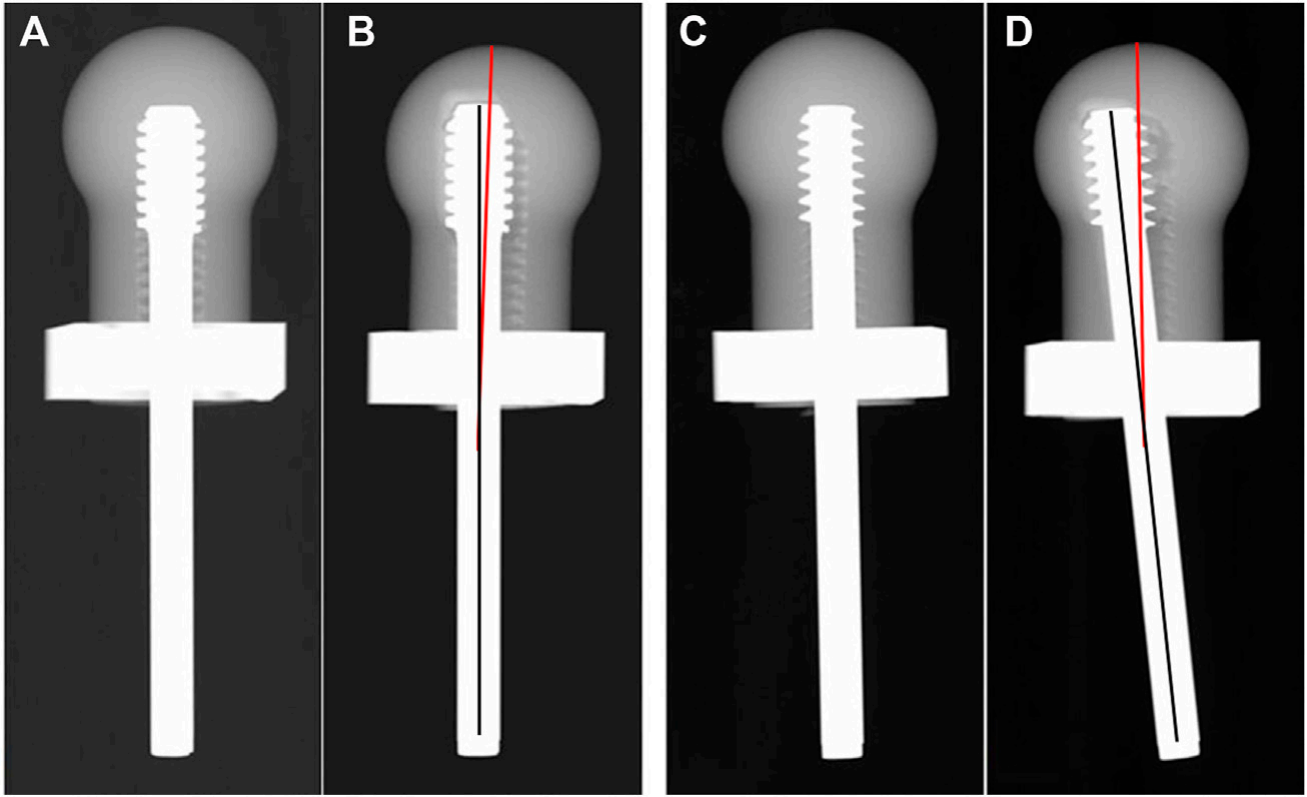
Images of X-ray scan pre-test (A,C) and post-test (B,D). The solid black line represents the axis of the screw, the solid red line represents the axis of the femoral head.
